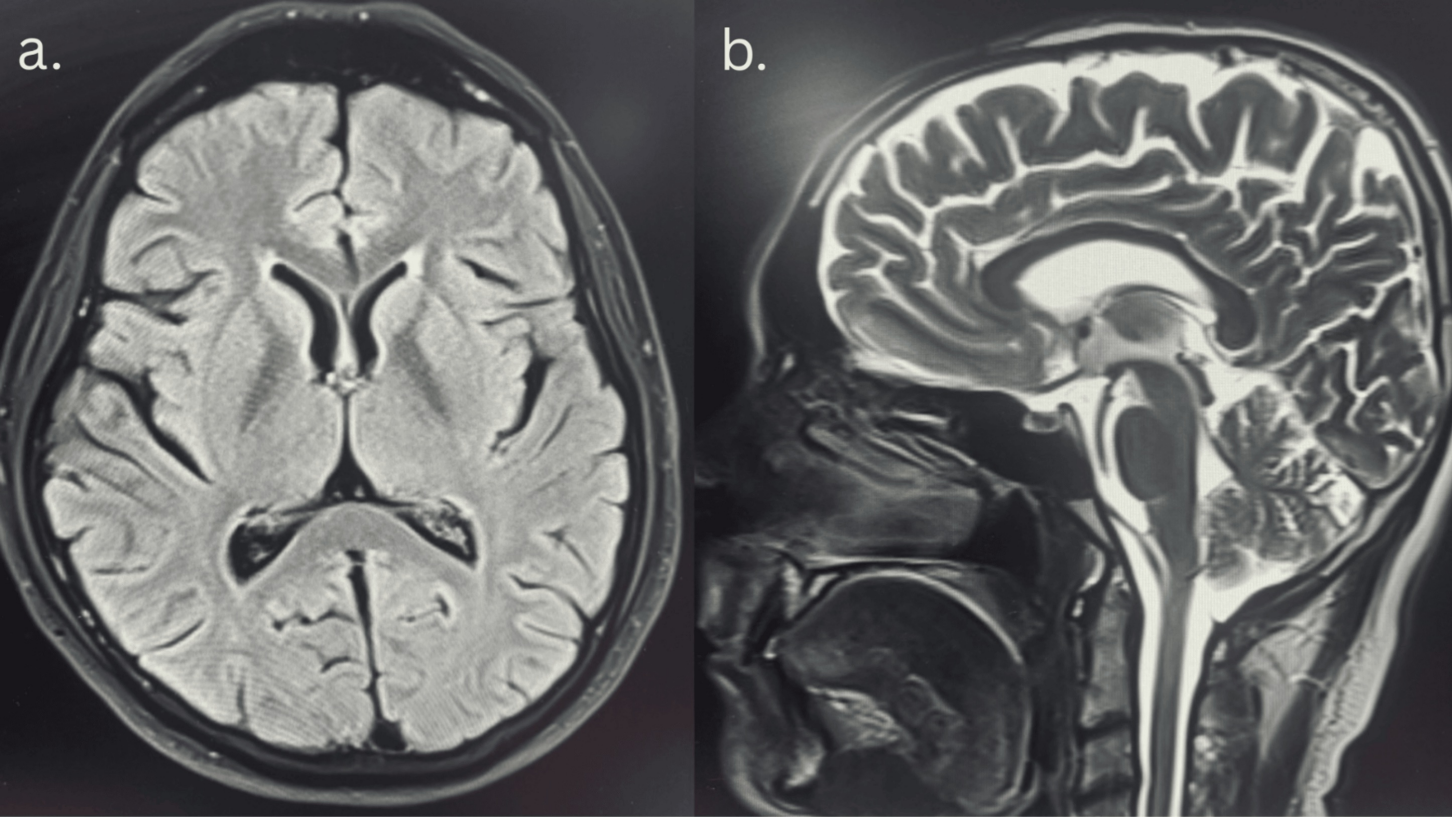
(a) MRI axial FLAIR and (b) sagittal T2-weighted (right) images of this patient with VGCC autoimmune encephalitis, showing no abnormal signal or enhancement. MRI, magnetic resonance imaging; FLAIR, fluid-attenuated inversion recovery; VGCC, voltage-gated calcium channel
